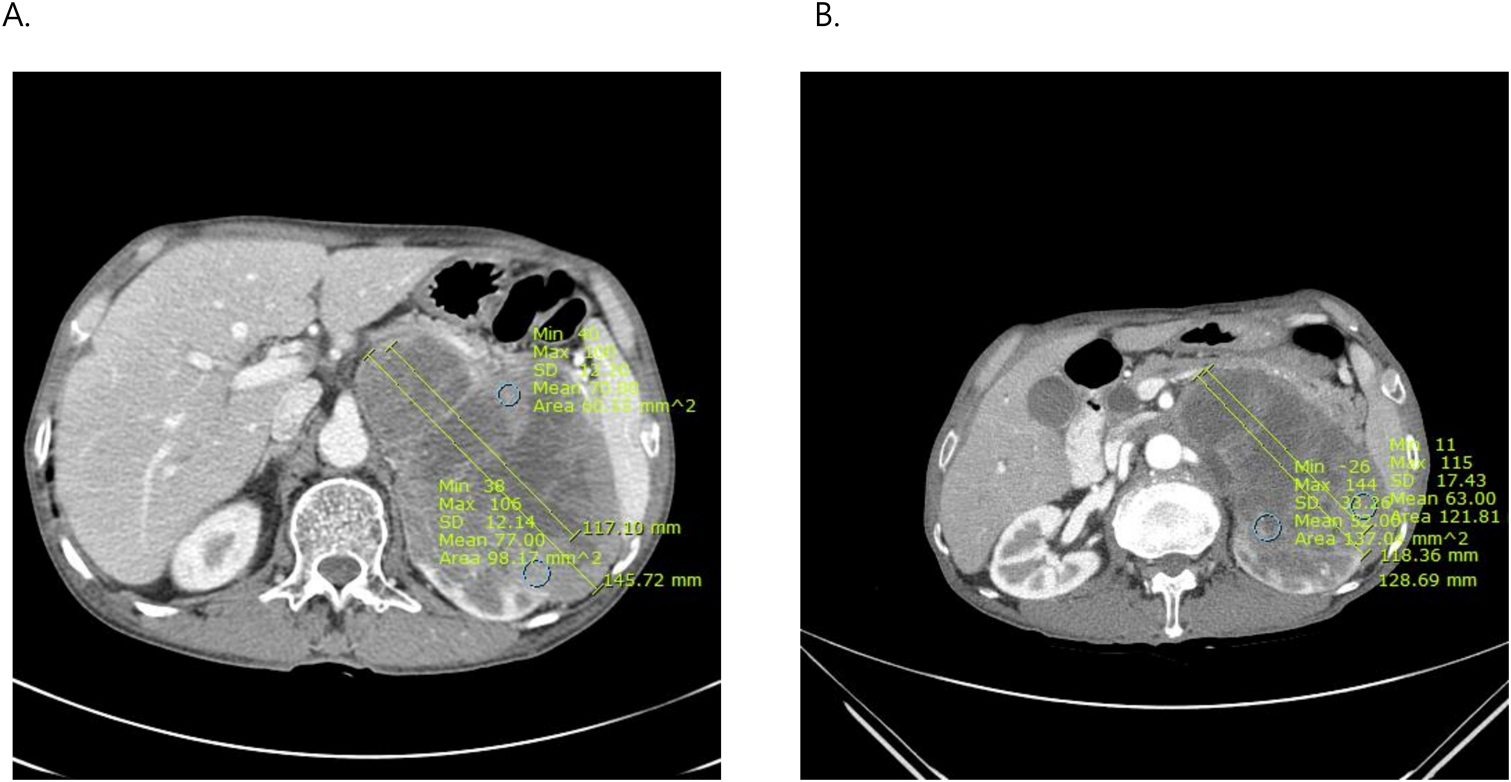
Representative changes of tumor size and necrosis size with attenuation on first follow-up contrast-enhanced computed tomography (CT) scans in metastatic renal cell carcinoma (RCC) patients with unresectable primary RCC. A. Axial CT image before sunitinib therapy showed a 14.5 cm-sized enhancing left renal mass with hilar invasion and presence of venous tumor thrombi. B. Compared to the CT image before targeted therapy, the CT image after 2-cycles of sunitinib showed that the tumor’s size had decreased (12.9 cm) with increased necrosis (11.8 cm) and decreased attenuation (from 77 Hounsfield units to 52 Hounsfield units).
